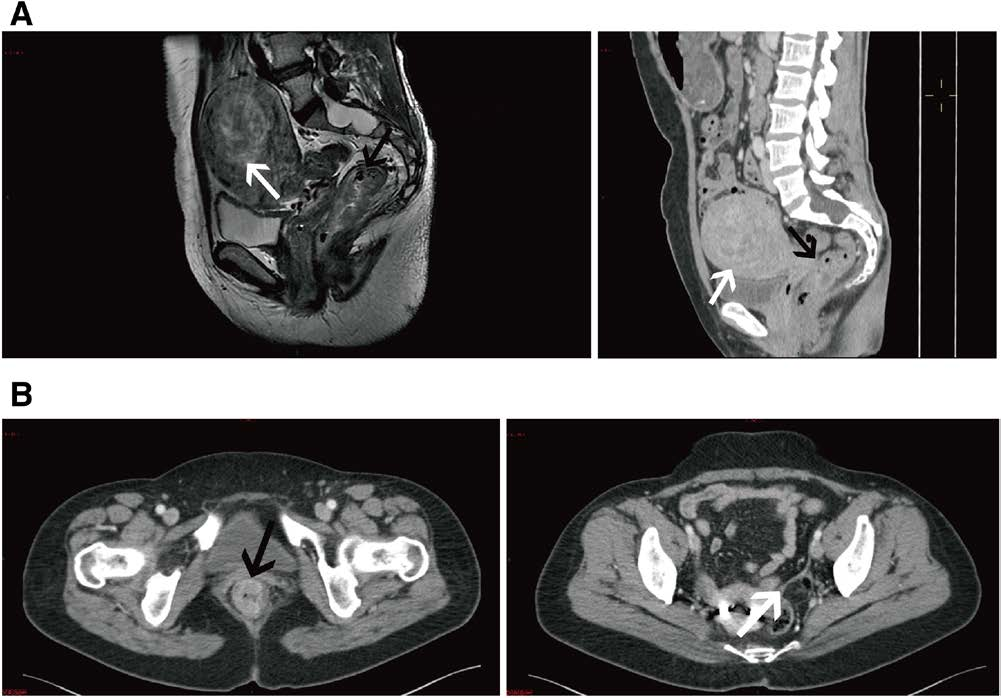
Pelvic incidentalomas included uterus and ovary incidentalomas. (A) A 52-year-old woman with rectal cancer (black arrow) and a 6.7 cm uterus fibroid (white arrows) on T2WI median sagittal MR and CT images. (B) A 50-year-old woman with rectal cancer (black arrow) and a 2.5 × 2 cm teratoma (white arrows) in the left ovary.
